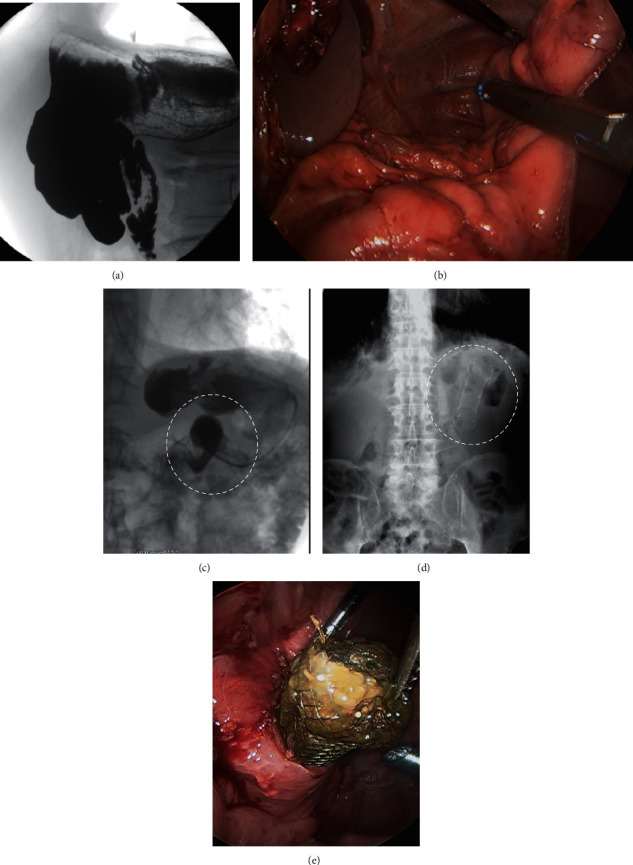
(a) Barium swallow: organoaxial gastric volvulus. (b) Laparoscopy: longitudinal gastrectomy with a very long staple line. (c) Fluoroscopy: alpha-loop in the distal stomach. (d) Abdominal plain film: the stent migrated into the proximal jejunum and is seen in the left upper quadrant. (e) Laparoscopy: the jejunum is opened, and the migrated stent is retrieved.
